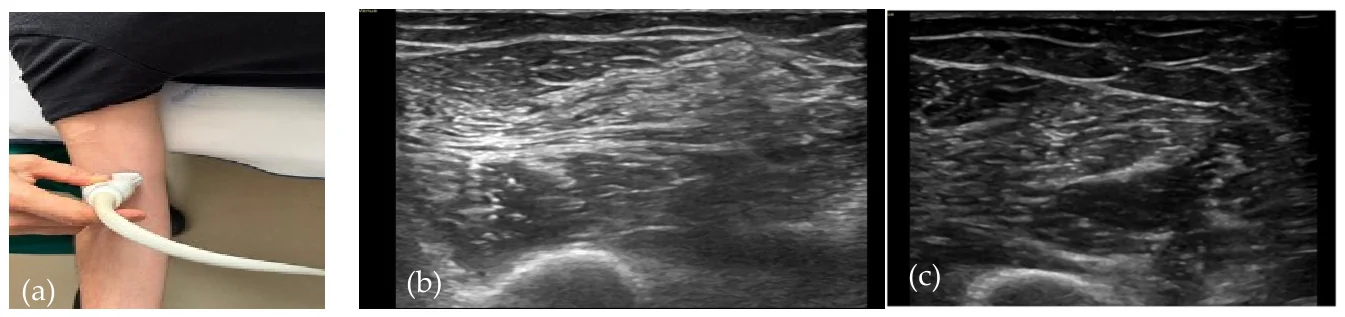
MSK US probe placement and brachialis muscle. Abbreviations: MSK US: Musculoskeletal ultrasound; T0: baseline assessment; T1: follow-up at 3 months; (a) Patient positioning and probe placement at the distal third of the arm in transverse orientation. (b) MSK US image of brachialis muscle at T0. Baseline ultrasound image demonstrating the brachialis muscle located between the humeral cortex and the overlying biceps brachii muscle. (c) MSK US of brachialis muscle at T1. Follow-up ultrasound image obtained at 3 months using the same acquisition protocol. Muscle architecture remained largely unchanged, with persistent heterogeneous echotexture and only subtle differences compared with baseline.
